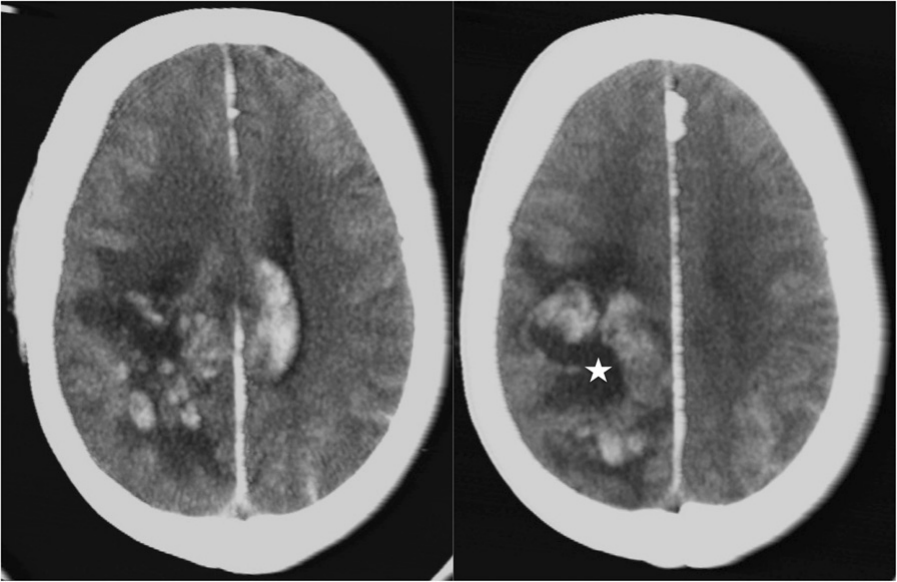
Axial CT scan without contrast medium injection. Spontaneous nodular hemorrhagic hyperdensity indicating establishment of an intraparenchymal hematoma with hypodense center (star). The right lateral ventricle is clear and the left is laminated. Note the presence of an associated subarachnoid hemorrhage
